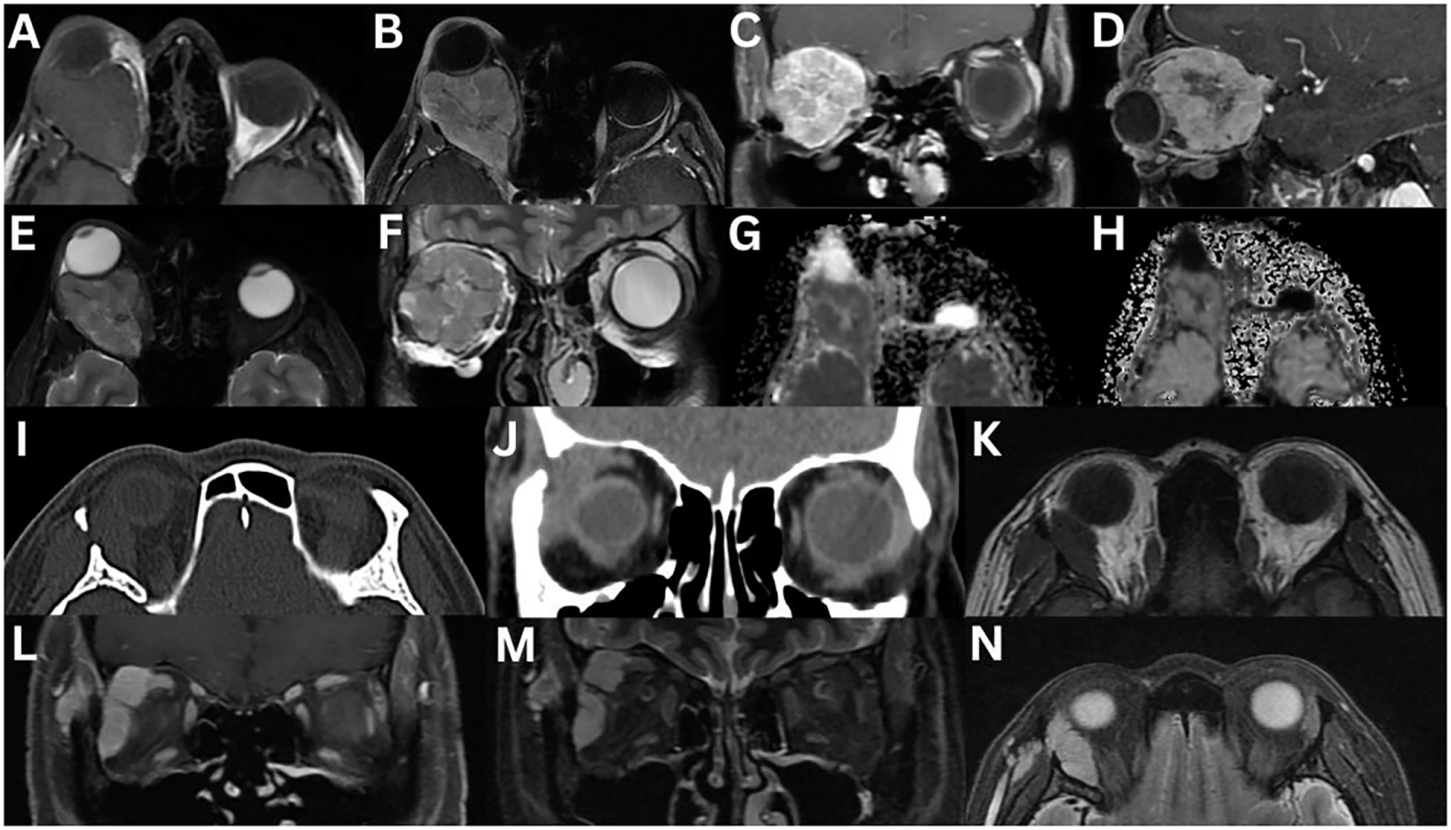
Adenoid cystic carcinoma of the right lacrimal gland (A–H). MRI T1-weighted axial (A), and fat-suppressed contrast-enhanced axial (B), coronal (C), and sagittal (D) sequences demonstrate an irregular, lobulated mass with evidence of central necrosis, infiltrating the extraocular muscles and the optic nerve sheath. The lesion is isointense to extraocular muscles on T1-weighted imaging and demonstrates a positive lateral wedge sign. T2-weighted axial (E) and coronal (F) sequences demonstrate a mass that is hyperintense to extraocular muscles on T2-weighted imaging. The axial ADC (G) and eADC (H) sequences demonstrate a dense, compact component with partial restricted diffusion. A 23-year-old with adenoid cystic carcinoma of the right lacrimal gland (I–N). CT axial (I) and coronal (J) sequences demonstrate bony orbital destruction. MRI T1-weighted axial (K), T1-weighted fat-suppressed contrast-enhanced coronal (L), coronal STIR (M), and T2-weighted fat-suppressed axial (N) sequences demonstrate a large, well-defined mass, that is isointense on T1-weighted imaging and hyperintense on T2-weighted imaging, relative to the extraocular muscles. Mild contrast enhancement is present.
